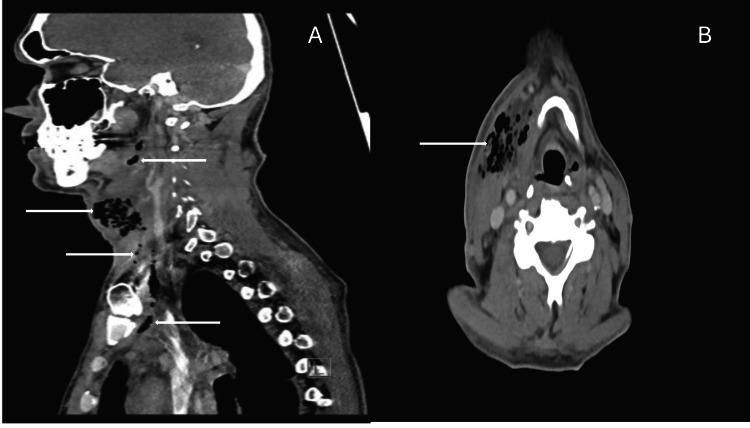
Initial CT scan views demonstrating cervical necrotizing fasciitis and descending necrotizing mediastinitis. (A) Sagittal contrast-enhanced CT scan view shows extensive gas formation (white arrows) throughout the right deep neck spaces, including the parapharyngeal, submandibular, and visceral spaces, with trace extension into the superior mediastinum. (B) Corresponding axial CT scan view from the same study further demonstrates multiple foci of gas (white arrow) within the deep tissues of the right neck, highly suspicious for rapidly progressing necrotizing fasciitis.
